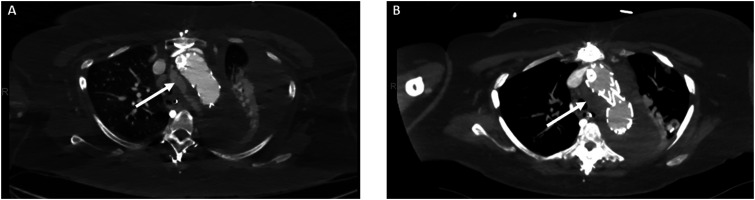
Postoperative day 1 CTA (A) with presence of type Ic endoleak (arrow) and adequate resolution after secondary intervention on postoperative day 5 (arrow) (B).
